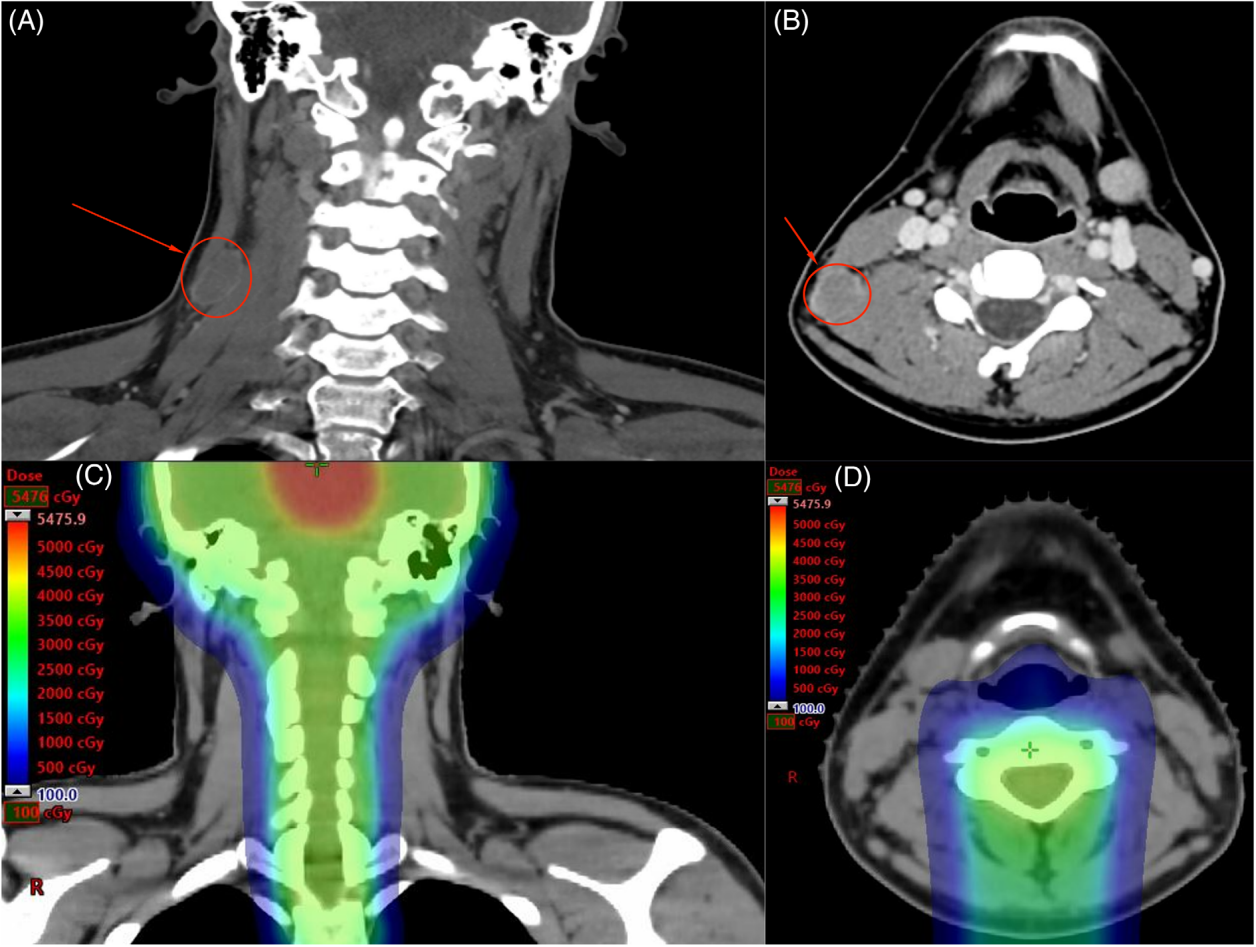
(A,B) After failing response to antibiotics, the enlarged level V right cervical lymph node was imaged with computed tomography. (C,D) This lymph node was located outside the target volume of the patient's prior proton therapy treatment
